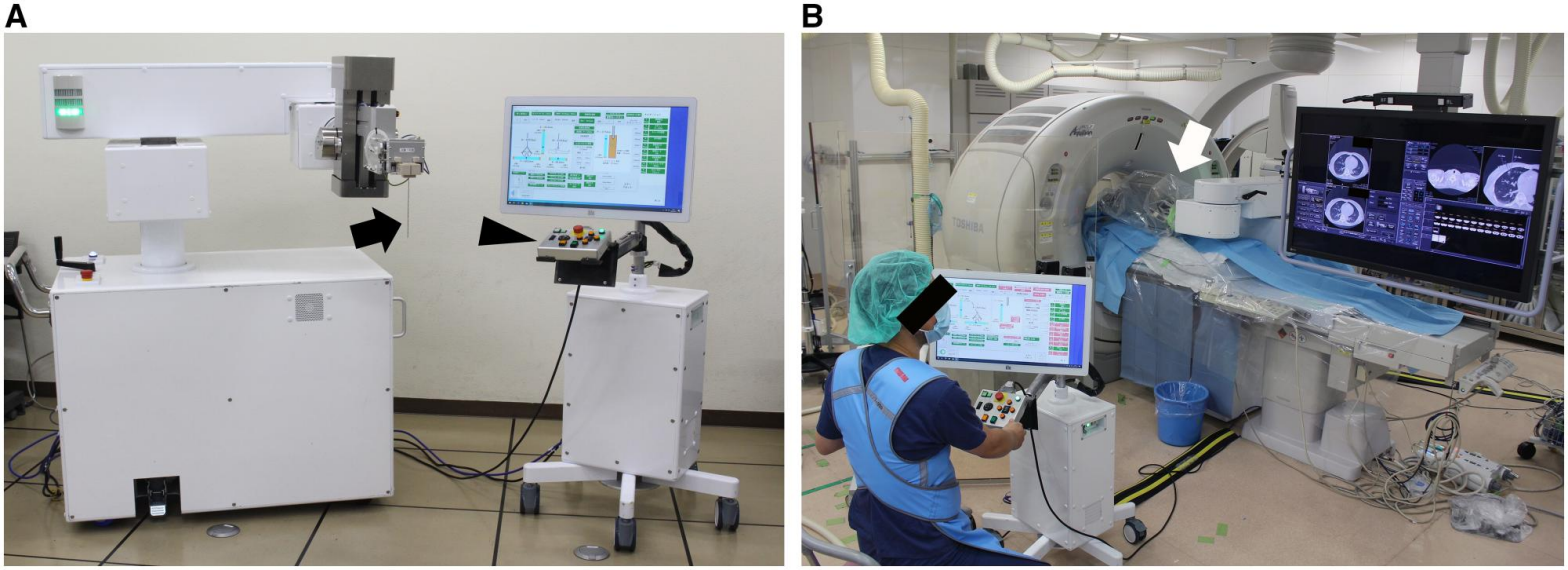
(A) The robotic system consisted of a robot (left) and a user interface (right). The needle (arrow) is attached to the end of the robotic arm. The interface includes a touch panel and a controller (arrowhead). (B) A photograph taken during robotic procedure. The physician sits behind a lead shield a few meters away from the CT gantry and operates the robot (arrow) through the interface under CT fluoroscopic guidance.
